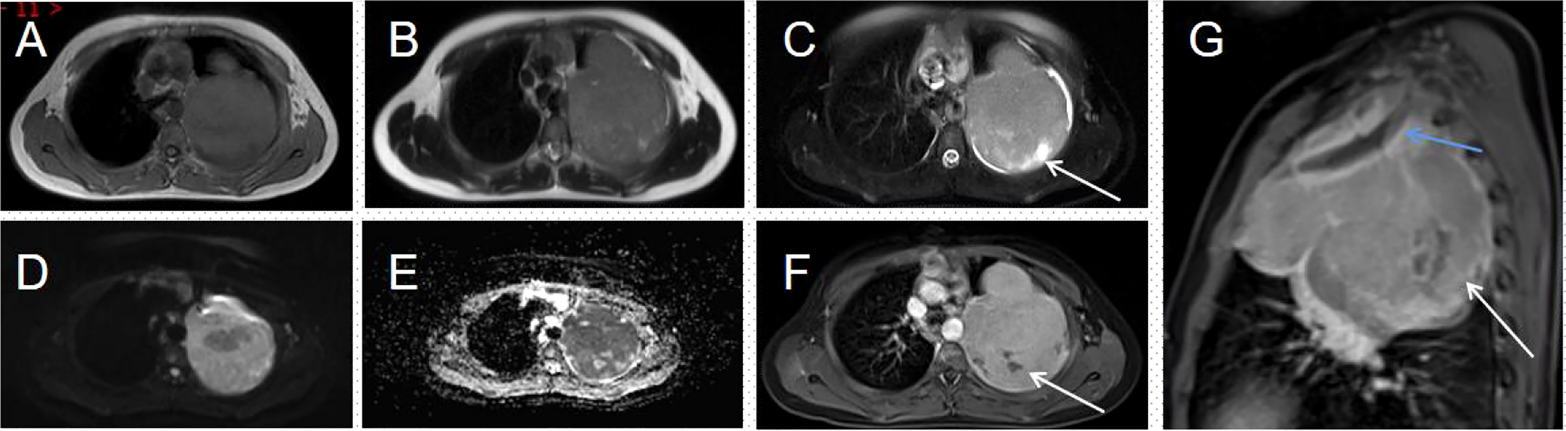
Magnetic resonance imaging (MRI) of the thorax (A–G). (A, B) An irregular soft tissue mass is seen in the upper part of the left thorax, showing a mixed signal dominated by iso-T1 and iso-T2 signals. (C) T2WI lipid suppression shows a heterogeneous slightly high signal; few long T2 signals are seen within the mass (arrow). (D) The solid part of the mass shows a distinct high signal on DWI. (E) This corresponds to a low signal on the ADC map. (F) The solid part of the lesion is heterogeneously enhanced; no enhancement is seen in the internal liquefied necrotic area (arrow). (G) The enhanced sagittal view reveals a bony destruction of the left first rib (blue arrow) and a lamellar area of non-enhancement within the mass (white arrow).
